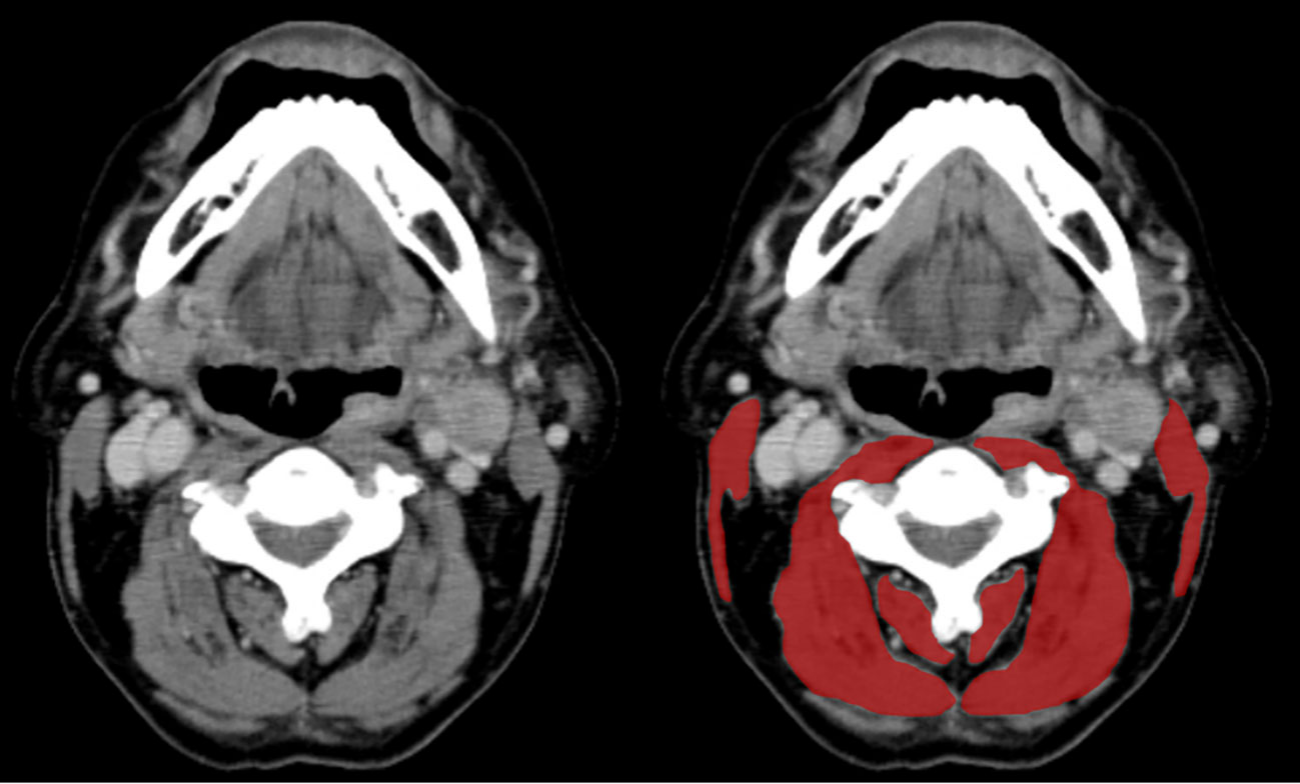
Paravertebral and sternocleidomastoid muscle area measurement at the level of the C3 vertebra. In the left axial CT slide, the muscle tissue is unsegmented. The right CT slide shows both the paravertebral and sternocleidomastoid segmented muscles in red.
